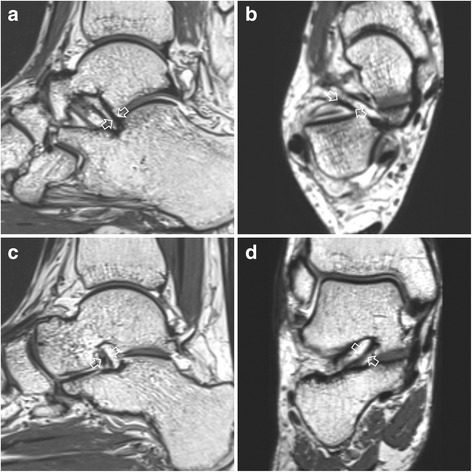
A 33-year-old woman with ankle sprain. Sagittal (a) and axial (b) isotropic 3D T2-weighted images demonstrating the thickness and width of anterior capsular ligament (open arrows), respectively. Sagittal (c) and coronal (d) isotropic 3D T2-weighted images demonstrating the thickness and width of interosseous talocalcaneal ligament (open arrows), respectively
